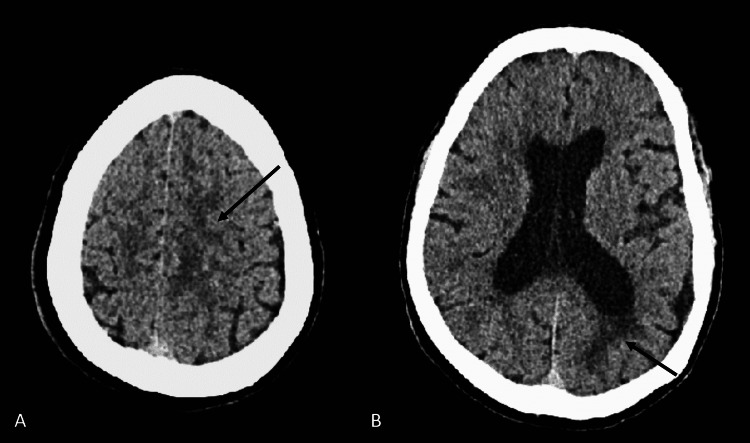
Computed tomography scan of the brain without contrast (A and B) Axial images showing mild to moderate hypodensities in left frontal and occipital lobes, suggestive of white matter disease (arrows).
